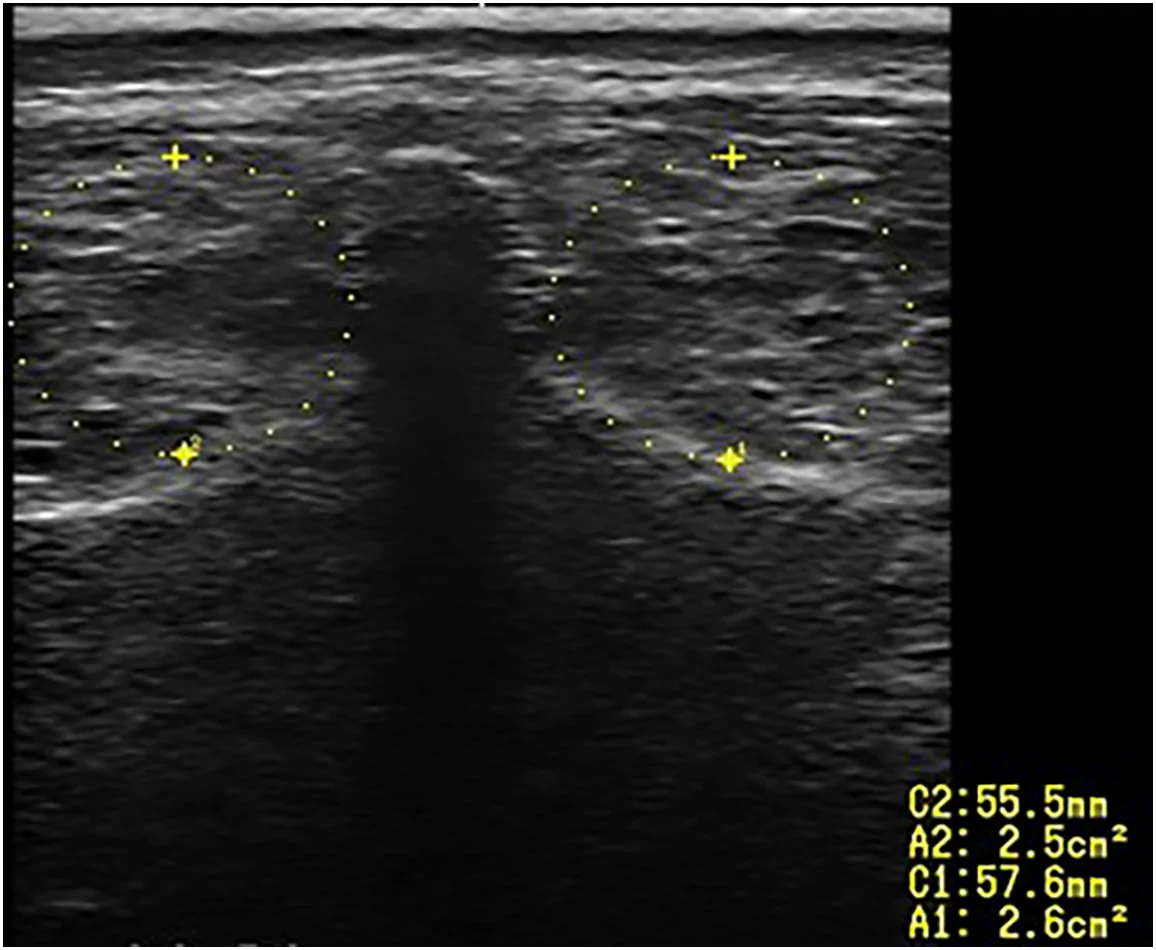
Ultrasound image of the cross-section of the Multifidus muscle. Created by the authors. Published under CC BY 4.0.
